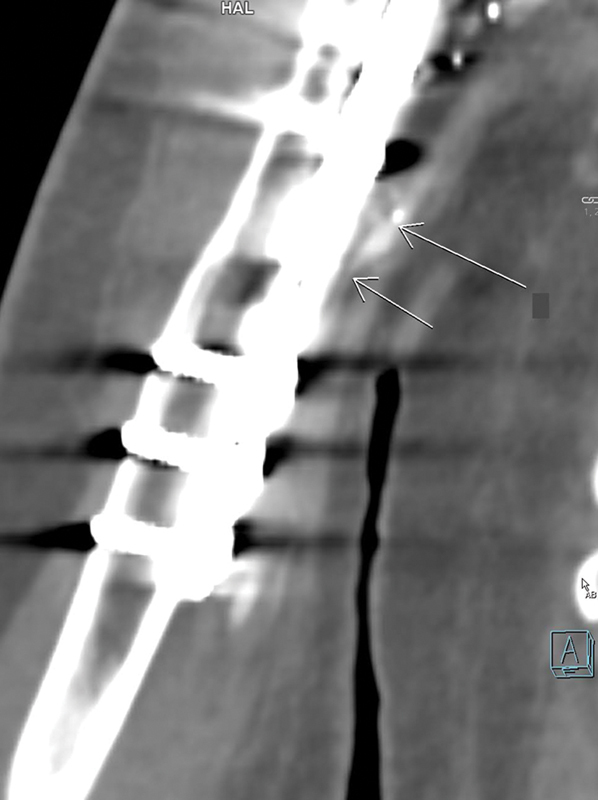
Computed tomography angiography showing patency of the vein graft along with patency of the flap's vessel.
